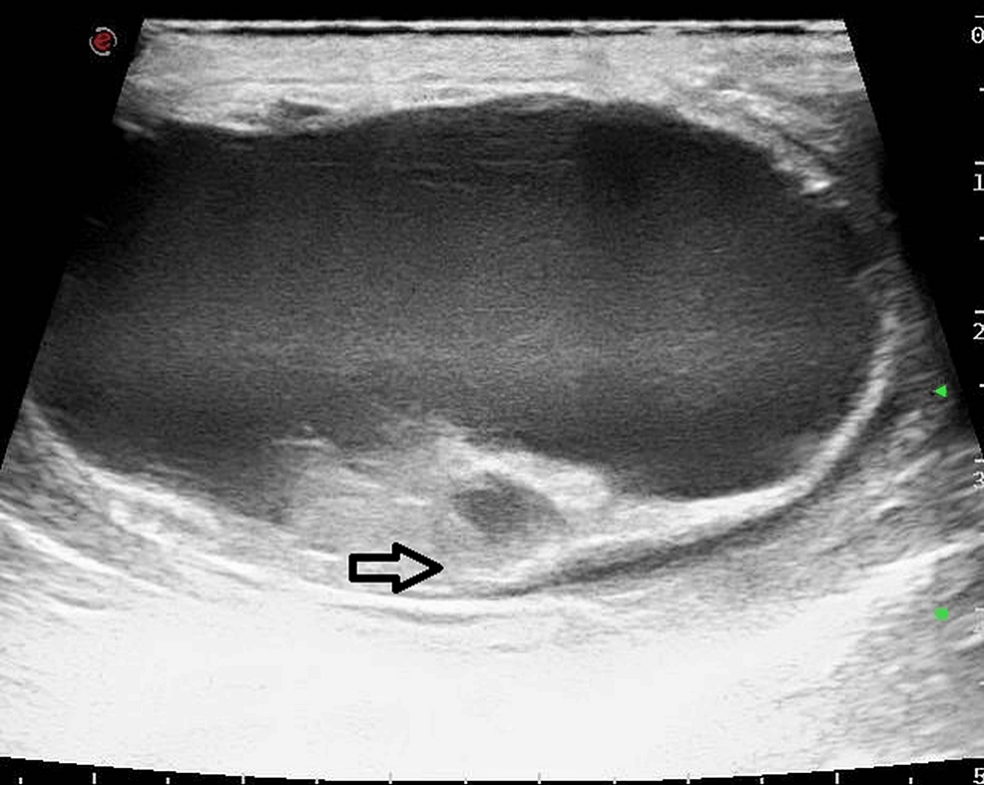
Longitudinal ultrasound view of the left epididymal cyst with fine internal echoes representing blood products.
